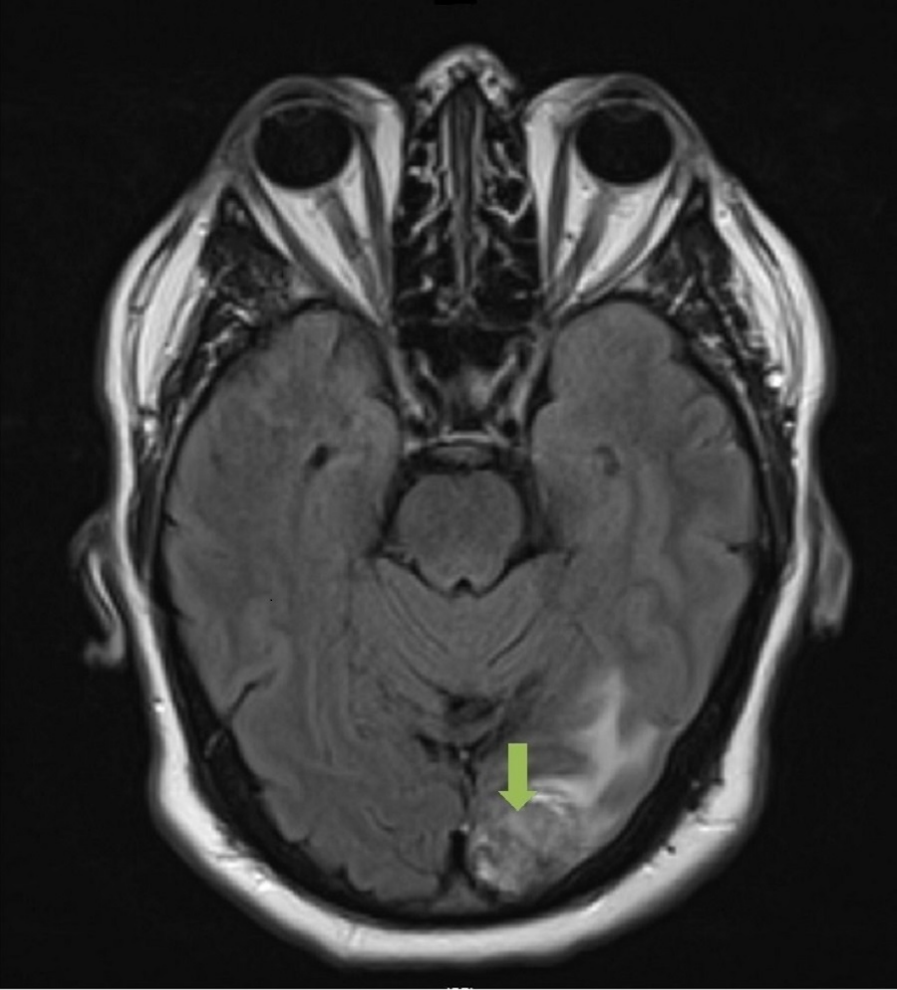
Preoperative MRI of the Head Axial slice. T2-weighted image that demonstrates a solitary 2.9 cm left occipital lobe adenocarincoma, metastatic from a lung primary.
